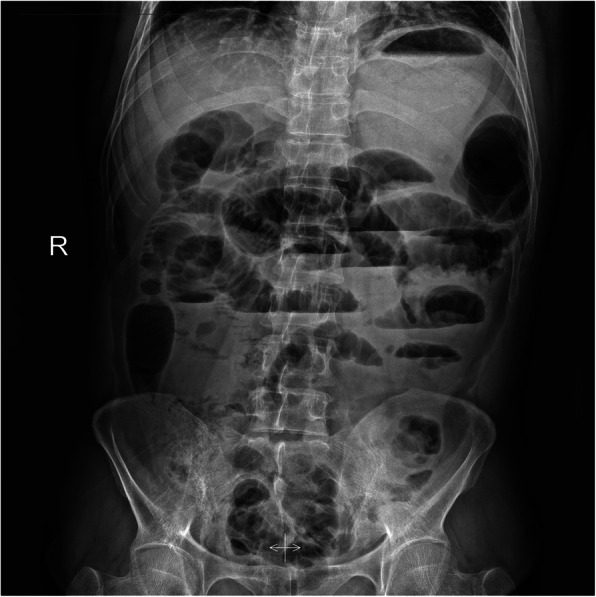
Erect abdominal x-ray at admission showing hugely dilated small bowel, with multiple air-fluid levels
